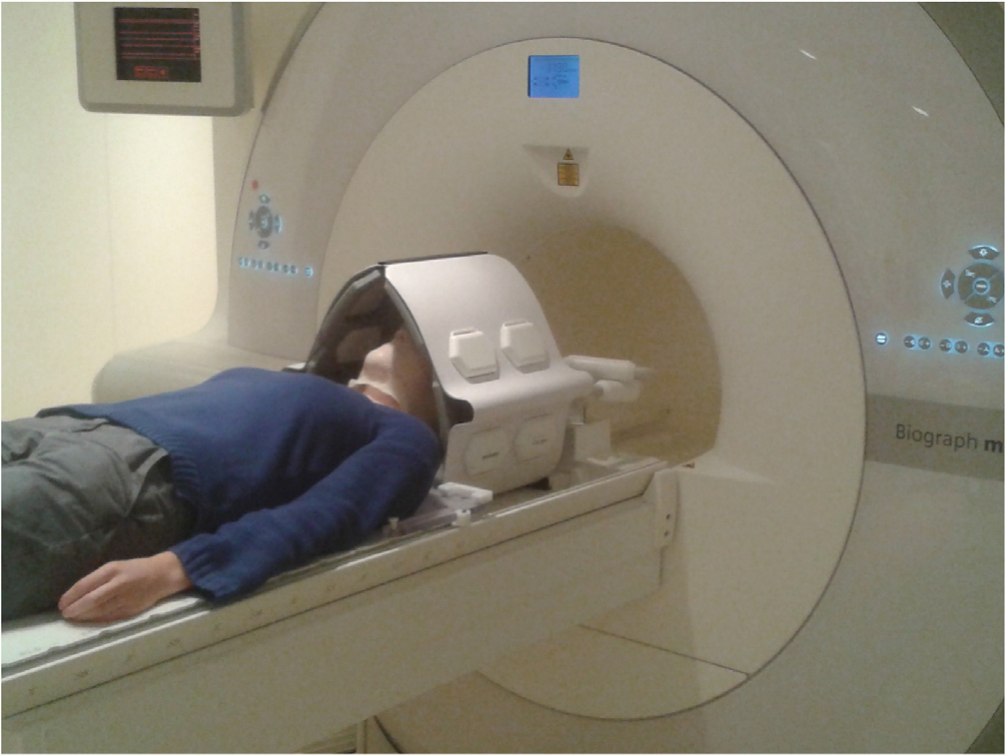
Dedicated positioning solution for MR imaging of head and neck cancer patients in RT treatment position. The components are a flat table top with an add-on for the fixation of a head and neck positioning mask, and coil holders to with flexible RF coils can be attached.
